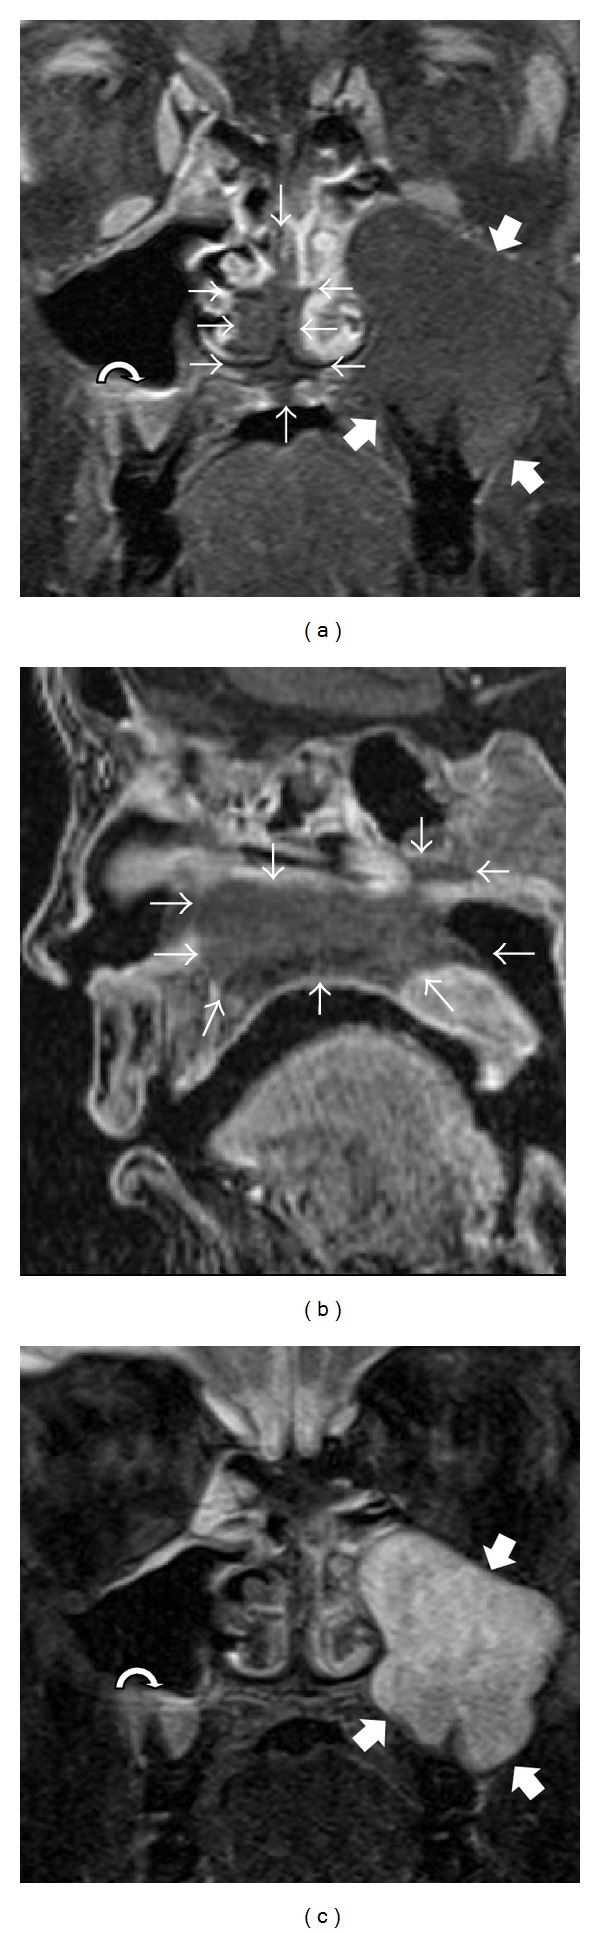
(a) Coronal postcontrast fat-saturated T1-weighted images (T1WI) and (b) sagittal postcontrast T1WI demonstrate sharply delineated hypoenhancement of the nasal septum and bilateral inferior turbinates extending inferiorly to the hard palate and superiorly to the inferior aspect of the sphenoid body (outlined by small arrows) without significant associated mucosal edema on STIR images (c). An odontogenic cyst centered on the crown of a left maxillary molar fills and expands the left maxillary sinus (thick arrows). A smaller maxillary odontogenic cyst is present on the right (curved arrow).
